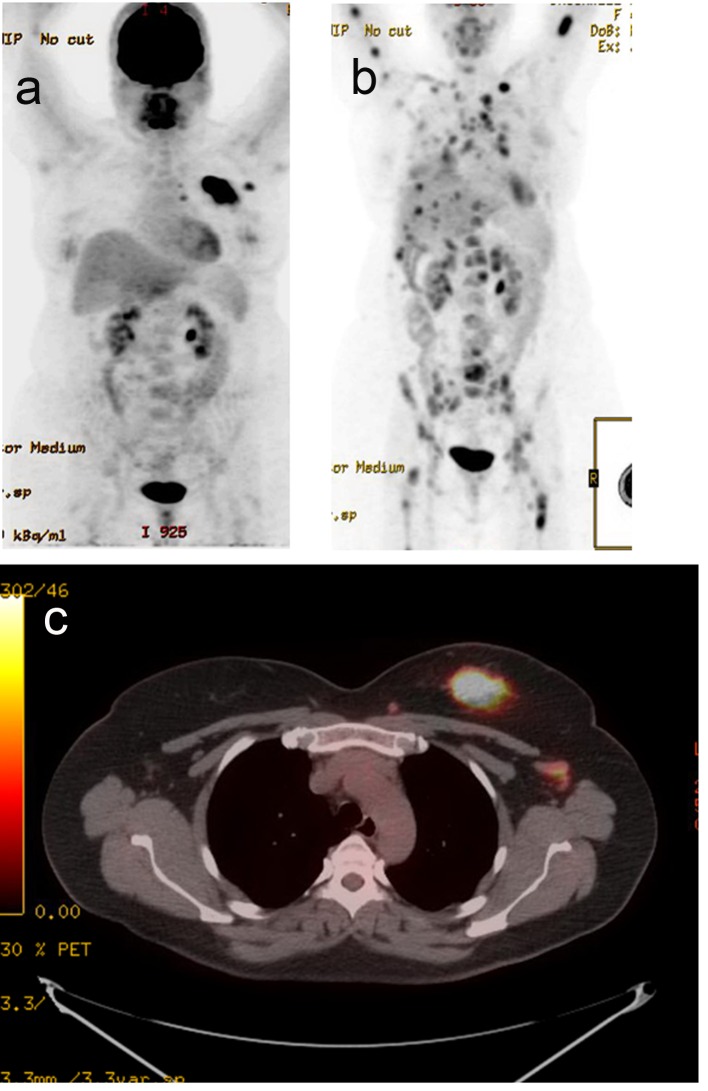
PET-CT. (a) Active left breast mass, left ax LN (September 2012). (b) Extensive distant metastasis (July 2013). (c) Active left breast mass (September 2012).
